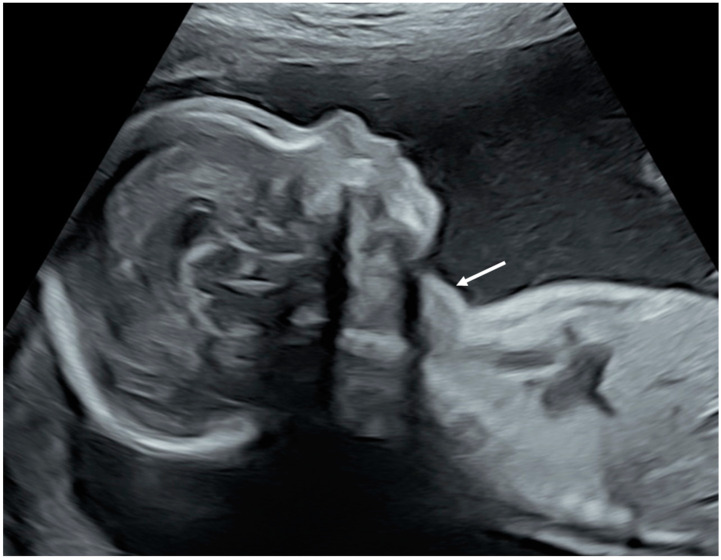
Sonographic view of fetal thyroid goiter from a sagittal plane (personal data from Bicetre Hospital Fetal Medicine Unit). The arrow points toward the fetal neck, which is distorted by a hyperthyroidic goiter.
